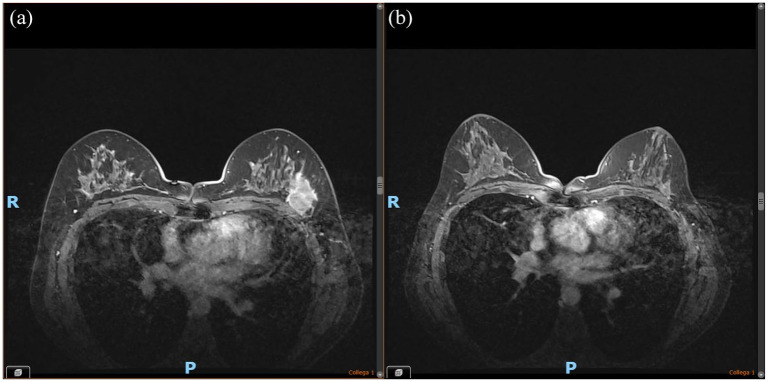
Breast MRI of Twin A. (a) Baseline. (b) Post-neoadjuvant therapy.
